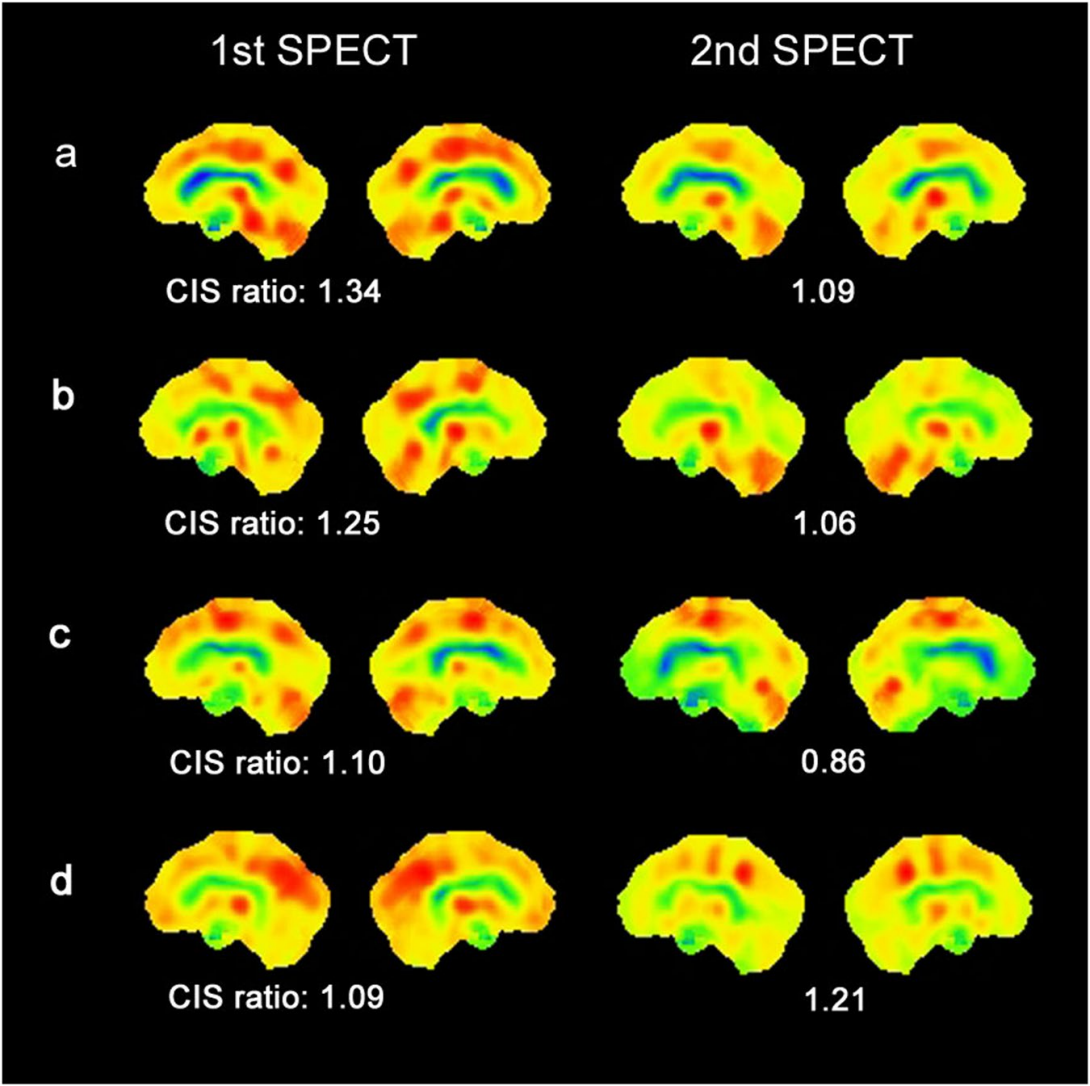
Temporal changes in CIS ratios in 3D brain perfusion images from 4 patients. Values below each brain image are CIS ratios. (a) Male patient with mild DLB aged 75 years with higher initial CIS ratio. The 2nd SPECT evaluation shows that CIS has become unclear, but CIS ratio remains over 1.00. MMSE scores at 1st and 2nd SPECT were 22 and 19, respectively. (b) Male patient with mild DLB aged 74 years with higher initial CIS ratio that became unclear two years later, although it remained over 1.00. MMSE scores at 1st and 2nd SPECT were 22 and 20, respectively. (c) Male patient with mild DLB aged 78 years with lower 1st CIS ratio. MMSE scores at 1st and 2nd SPECT were 23 and 18, respectively. (d) Female patient aged 76 years with prodromal DLB. At 1st SPECT, CIS was unclear but became obvious at 2nd SPECT. MMSE scores at 1st and 2nd SPECT were 25 and 21, respectively.
